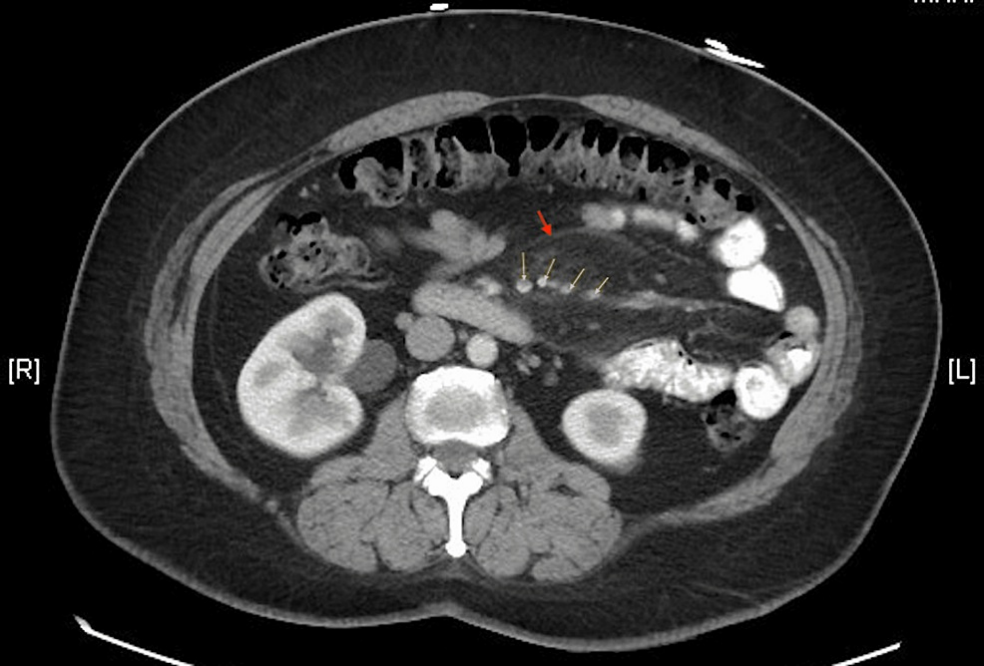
Coronal abdominal CT with contrast demonstrating well-defined mass in mesentery with a pseudocapsule (red arrow). Contained within is focally enhancing mesenteric fat (yellow arrows)
